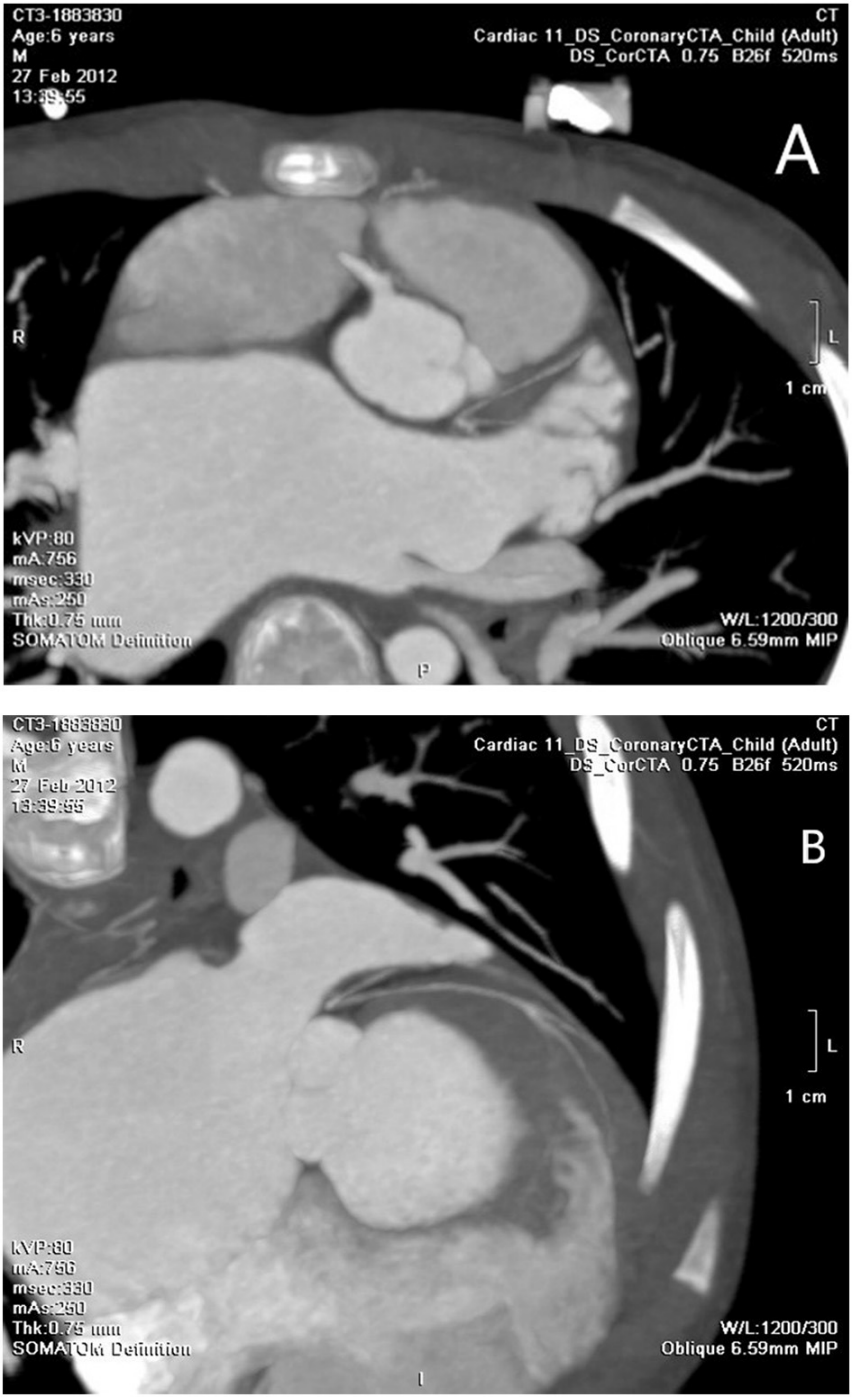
(A) Cardiac CTA: No left coronary artery opening in the left coronary sinus. (B) Cardiac CTA: hypoplasia of left anterior descending coronary artery and left circumflex coronary artery.
